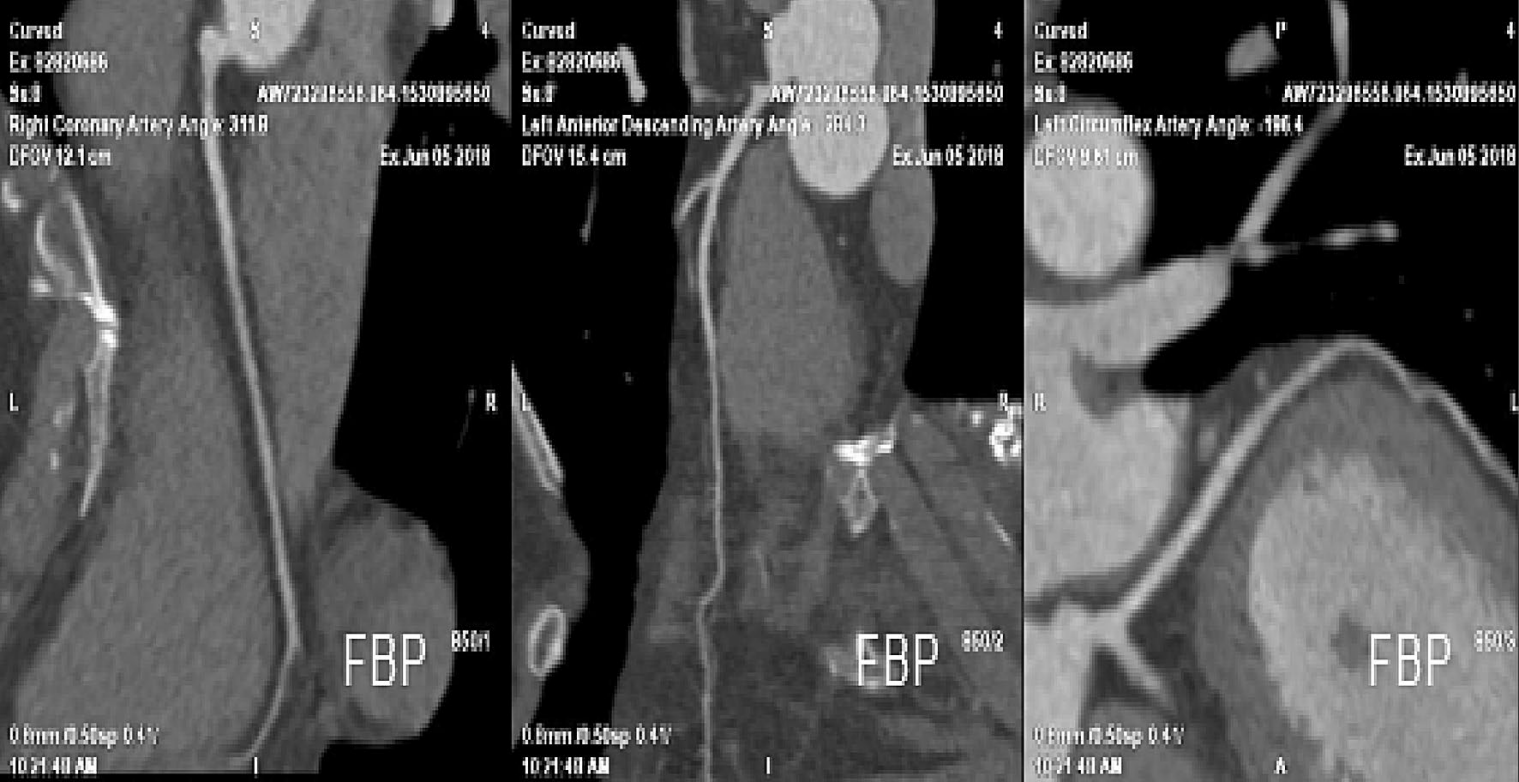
Representative image of a 70-year-old female patient, body surface area (BSA) 1.58 m^2^, body mass index (BMI) 27.27 kg/m^2^, heart rate (HR) 96 bpm, obtained using 40 mL contrast agent injected at a flow rate of 3.5 mL/s on a Flash computed tomography scanner.
